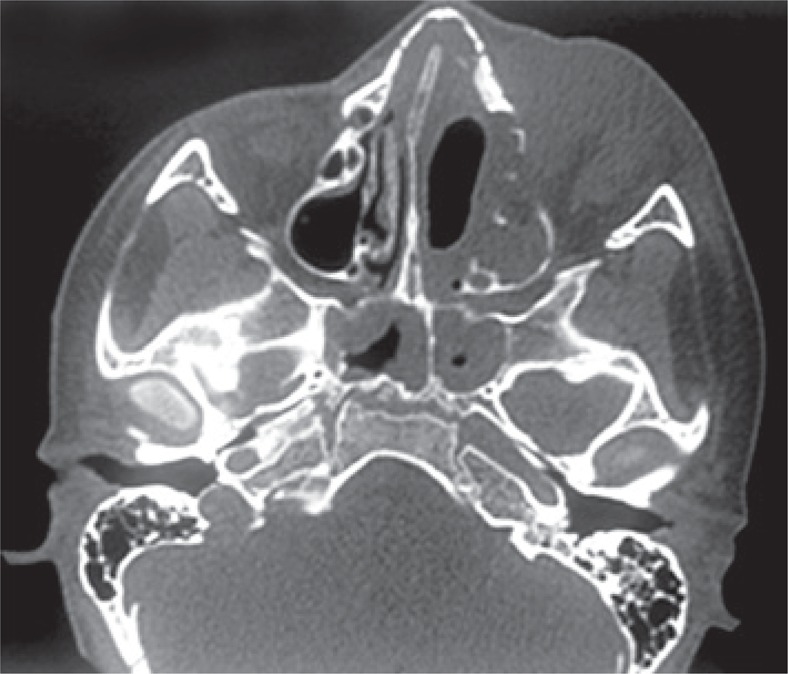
Computed tomography of the face sinuses. Signs of persistence of lesion in edges of surgical sites toward left pterygopalatine fossa, remaining antral cavities to the left and large orbital component also to the left, which infiltrated pre and post-septal plans and the lachrymal apparatus
